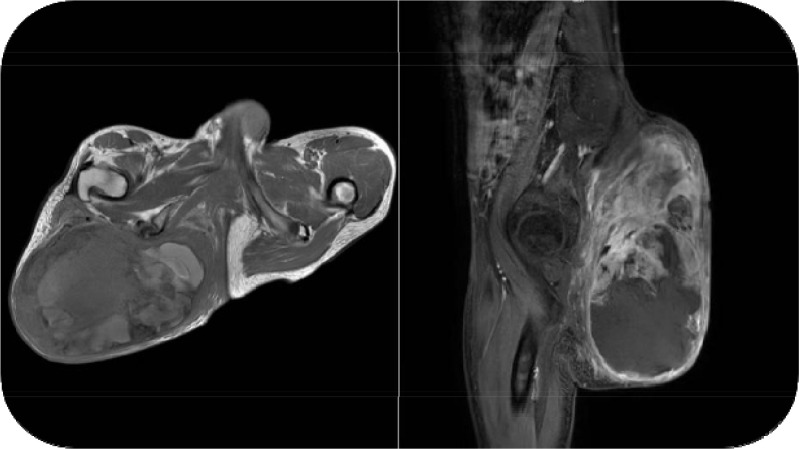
Preoperative axial and sagittal MRI views of the buttocks: A neoplastic lesion was observed in the subcutaneous tissue of the right buttock, with a maximum cross-sectional size of approximately 16.6 cm × 12.1 cm × 21.2 cm.
